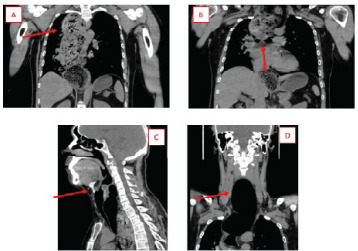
A:* Coronal computed tomography (CT) chest demonstrating megaesophagus with impacted food products. *B:* Coronal CT chest demonstrating compressed carina with deviation of mid-trachea to the left. *C:* Sagittal CT neck demonstrating arrowing of trachea and anterior displacement of epiglottis. *D:* Coronal CT neck demonstrating dilated, air-filled esophagus.
